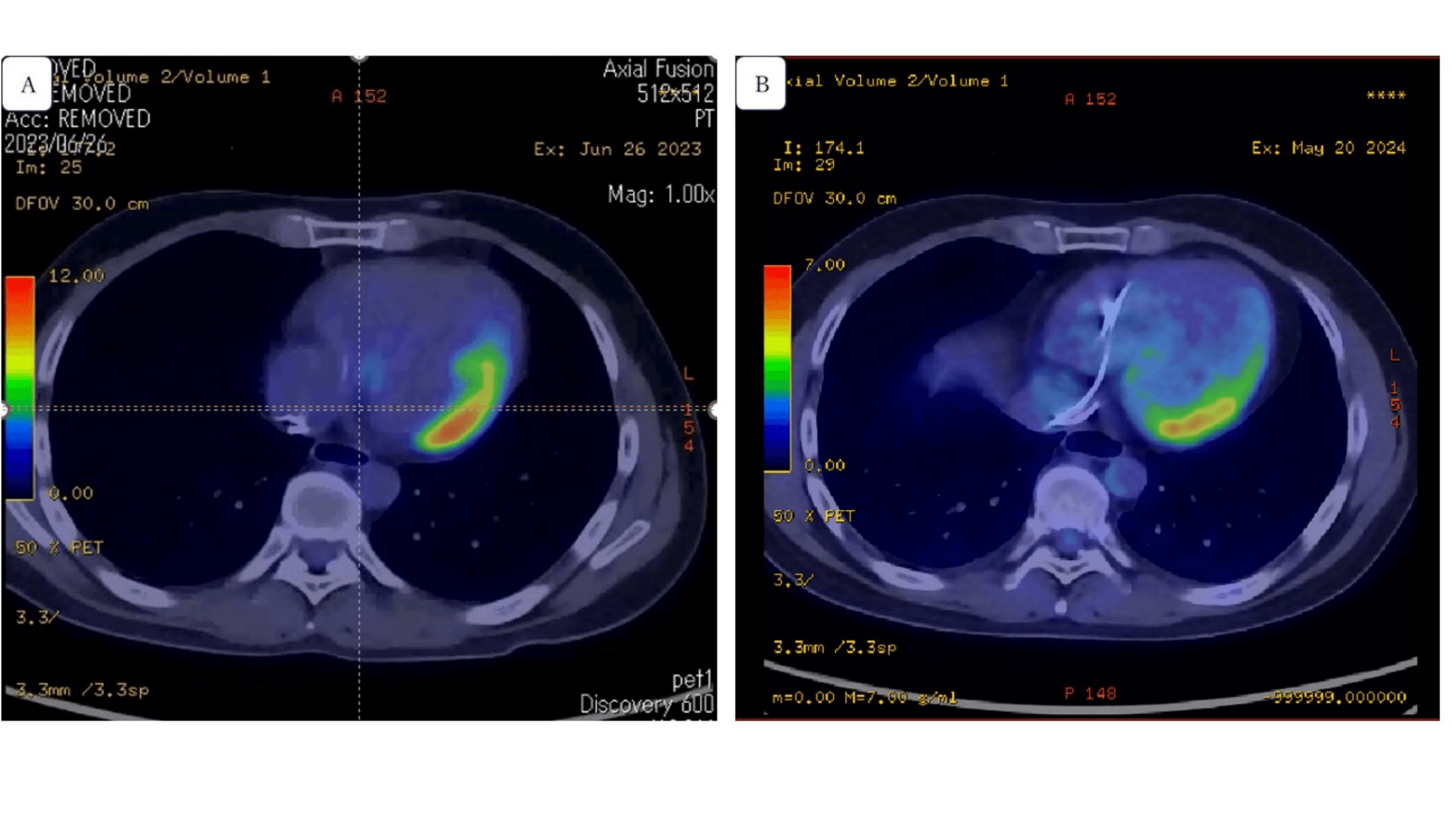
FDG-PET/CT images (A) The images show high uptake in the lateral wall (SUV max = 11.13). (B) Ten months later, follow-up images show less uptake in the lateral wall (SUV max = 5.14). FDG-PET/CT, fluorodeoxyglucose-18-positron emission tomography/computed tomography; SUV, standardized uptake value
